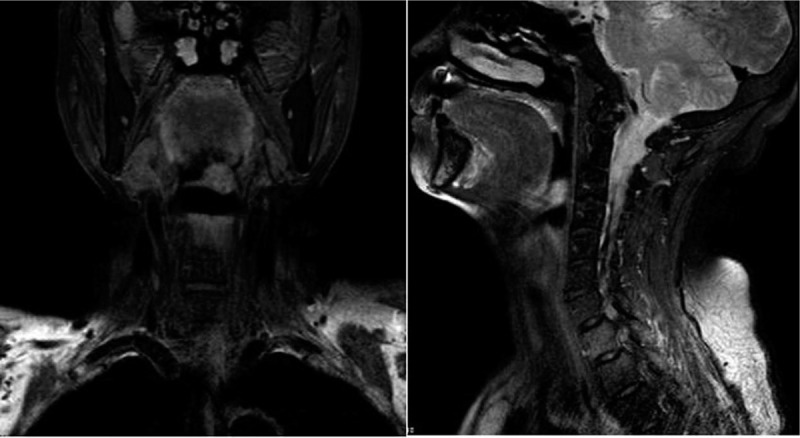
Magnetic resonance imaging shows a non-homogeneous soft tissue mass arising from the left epiglottis.
